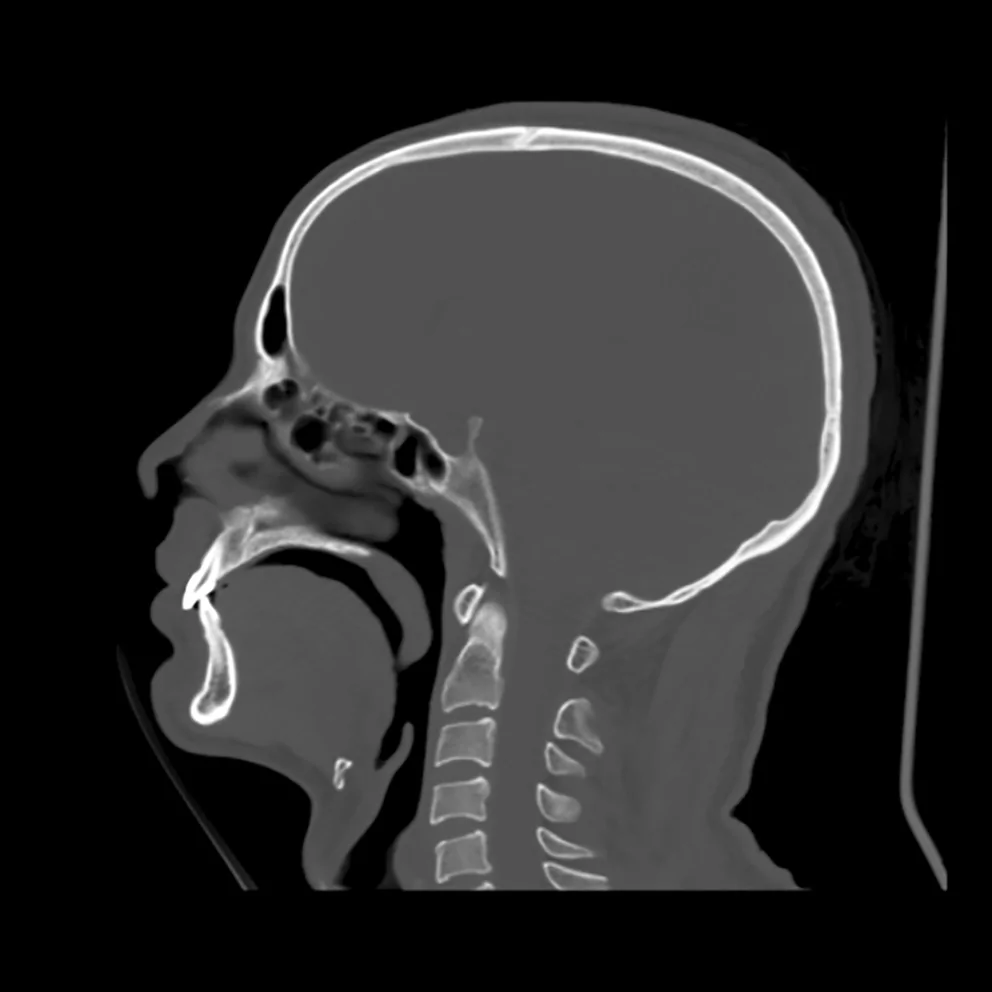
Non‐contrast sagittal CT images of the cervical spine demonstrating normal findings.
